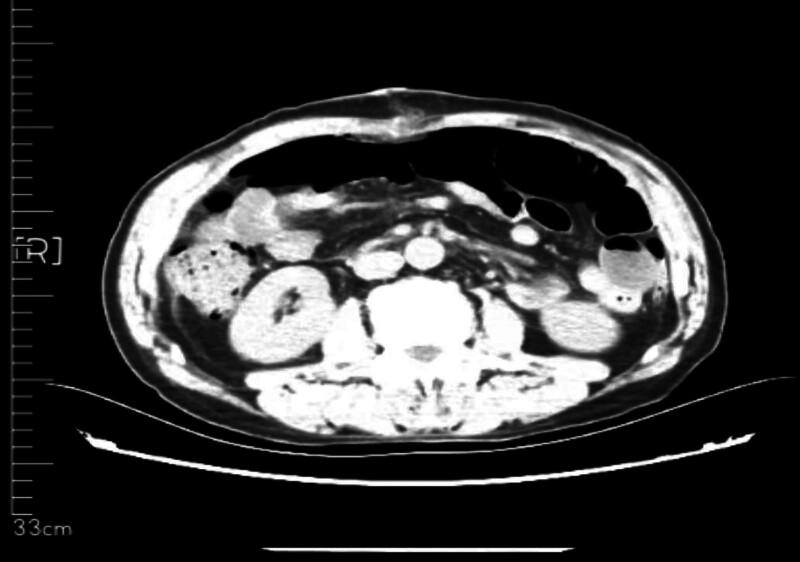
Postoperative CT shows that the liposarcoma in the abdominal cavity has been completely removed.
